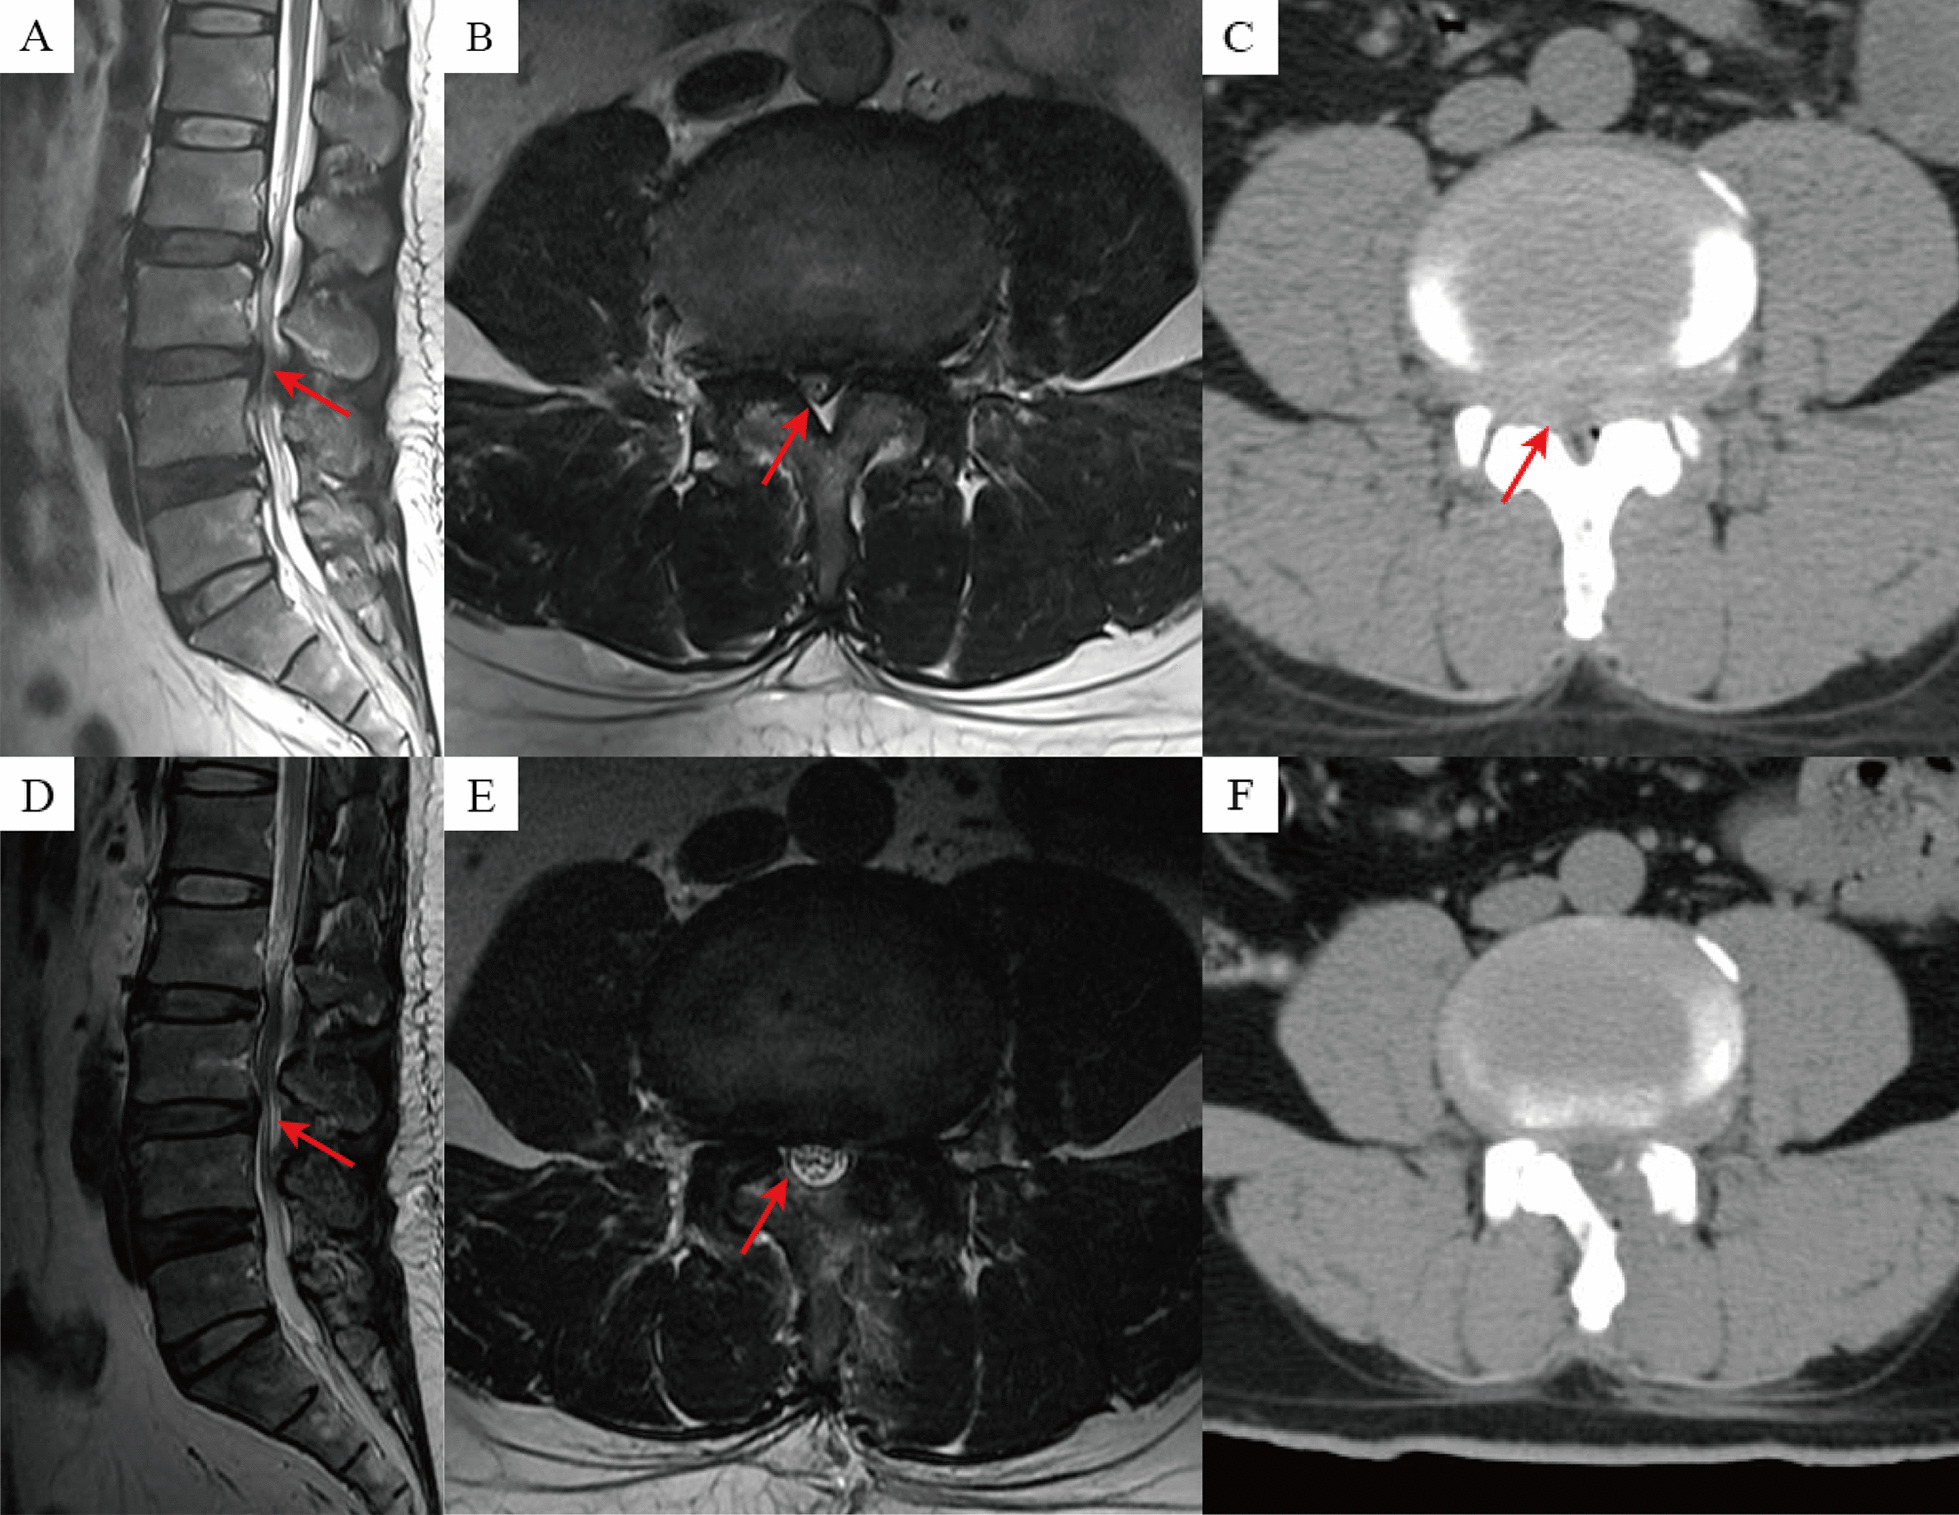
Images from a patient treated with OSE technique. A–C Preoperative MRI and CT showed L3-4 segment disc herniation with spinal stenosis; D–F Postoperative MRI and CT showed removal of the disc herniation, and adequate decompression of the spinal canal
